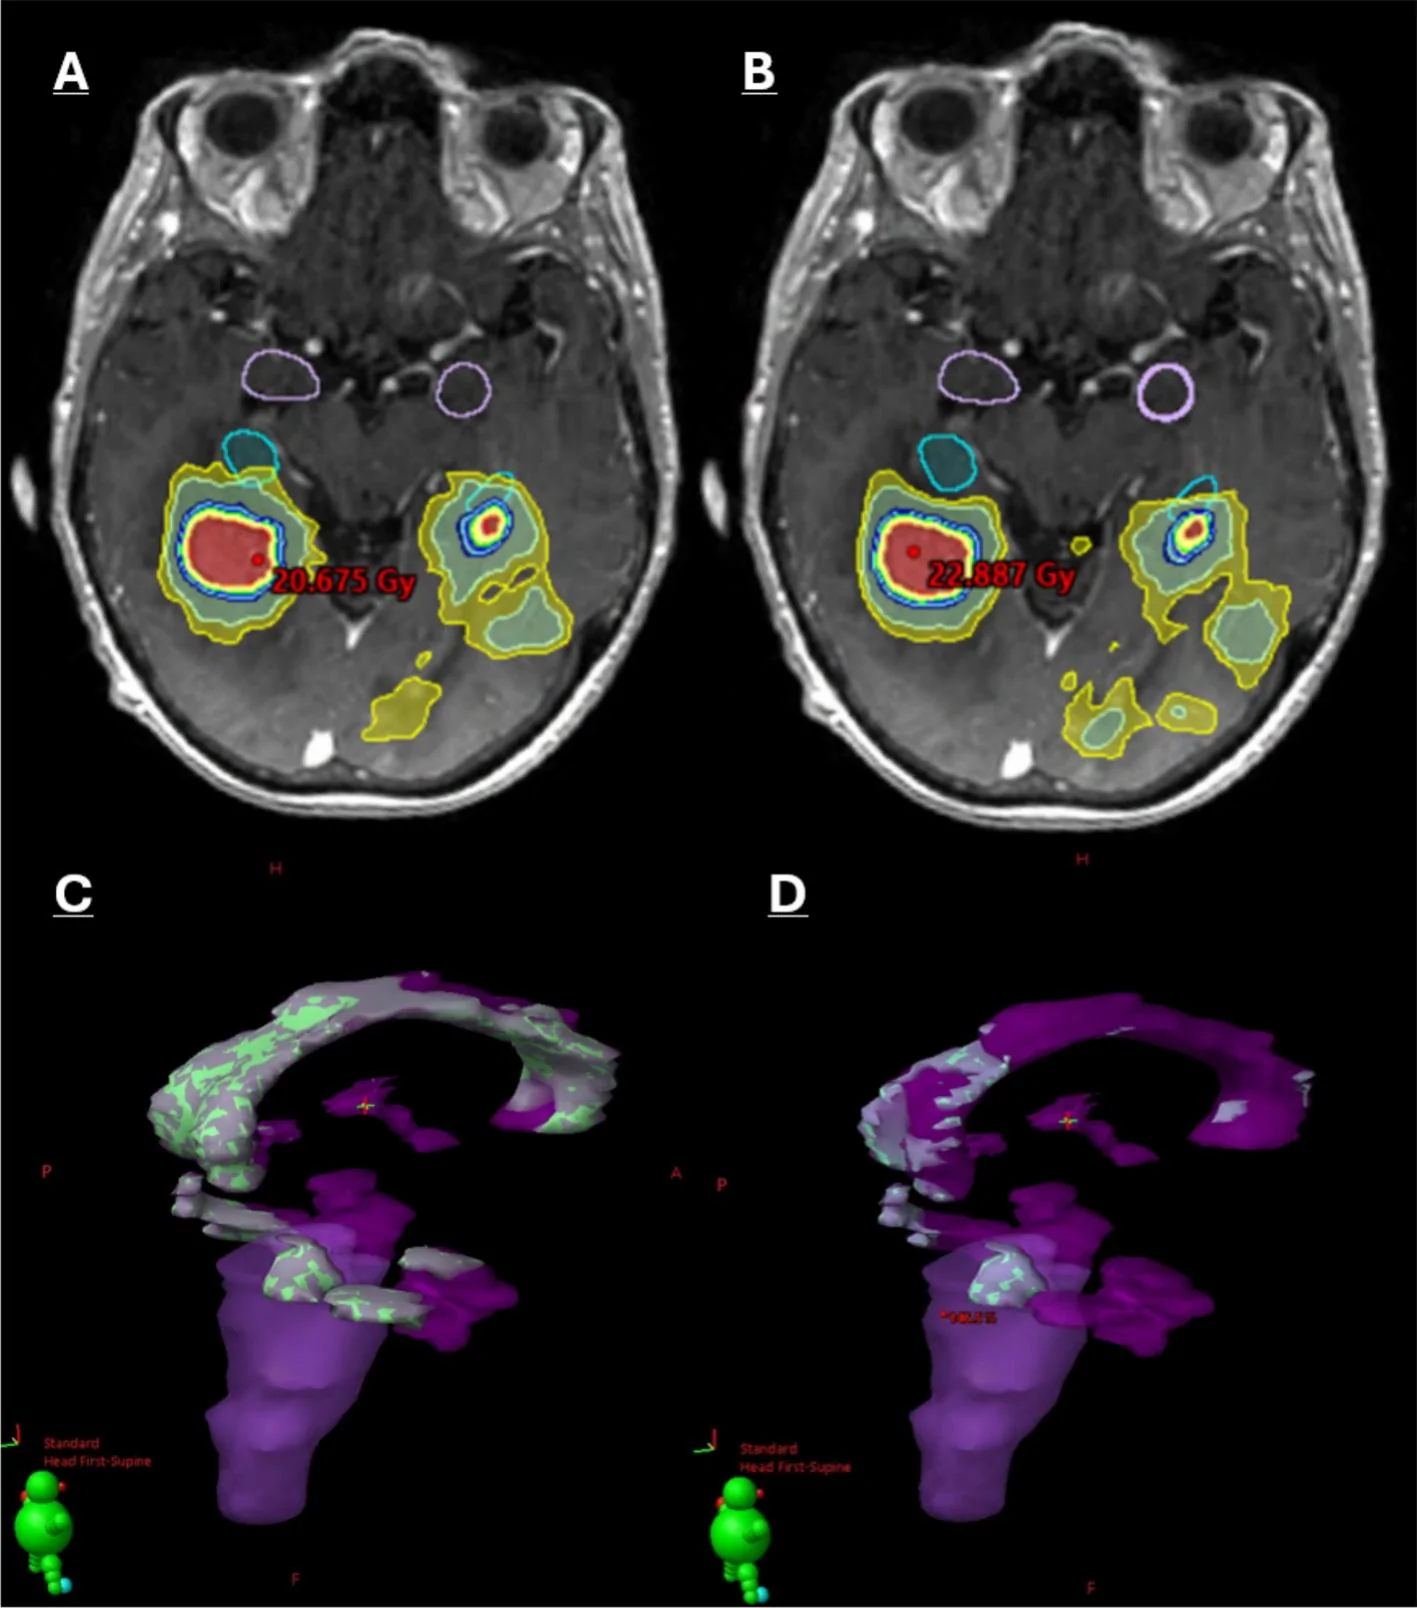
Color-washed FSPGR post-contrast MRI fused axial slices of a patient with several metastases in proximity with the amygdala (lavender) and hippocampi (cyan) in the original treatment plan (A) and SMART replanned treatment (B). Prescription dose was 16 Gy. The 6.65 Gy isodose line is displayed in yellow; this line represents the RTOG 0933 hippocampal dose constraint converted to a biologically effective dose using an α/β of two. 3D visualization of overlap of 4 Gy dose cloud (green) with the MC in the clinical plan (C) and SMART plan (D). (For interpretation of the references to colour in this figure legend, the reader is referred to the web version of this article.)
